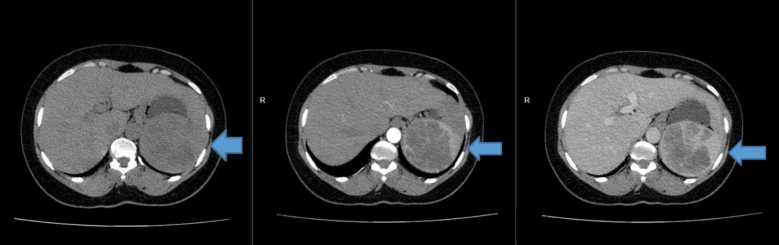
Contrast-enhanced axial CT scan images of the abdomen with the arrow showing a heterogeneous hypodense ill-defined vascular lesion within the splenic parenchyma.
